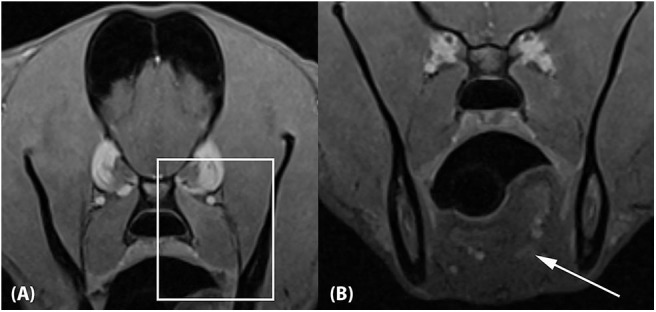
Approximately 10-week recheck MRI examination of the head. (A) Transverse T1-weighted Volume Interpolated GRE images with fat saturation (“VIBE”) at the level of the previous medial pterygoid muscle lesion and (B) the previous tongue base lesion. The lesions have mostly resolved, with mild residual contrast enhancement noted associated with the dorsal aspect of the left medial pterygoid muscle (A; box) and the tongue base (B; arrow). The left medial pterygoid muscle is mildly decreased in size compared to the right.
